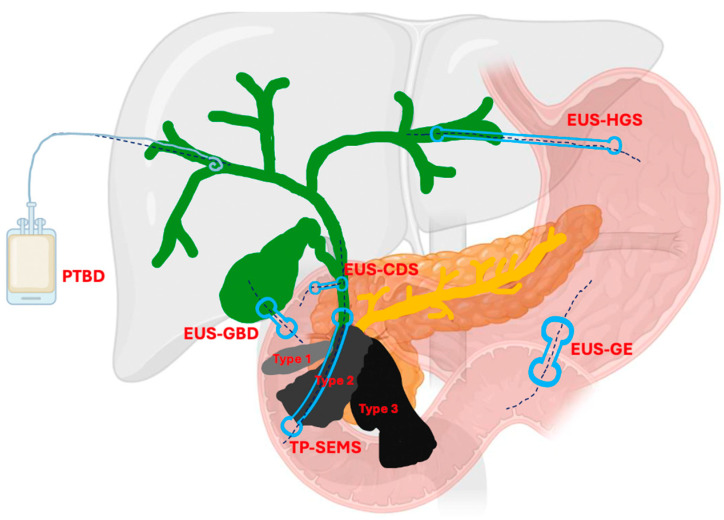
Management of malignant double obstruction (MBO). PTBD, percutaneous transhepatic biliary drainage. EUS-GBD, endoscopic ultrasound-guided gallbladder drainage. EUS-CDS, Endoscopic Ultrasound-Guided Choledochoduodenostomy. TP-SEMS, Transpapillary Self-Expandable Metal Stent. EUS-GE, Endoscopic Ultrasound Gastroenterostomy. EUS-HGS, Endoscopic Ultrasound-Guided Hepaticogastrostomy. Type 1, type 2, and type 3 duodenal stenosis according to Mutignani M et al. classification [83]. The copyright of the image belongs to the authors.
